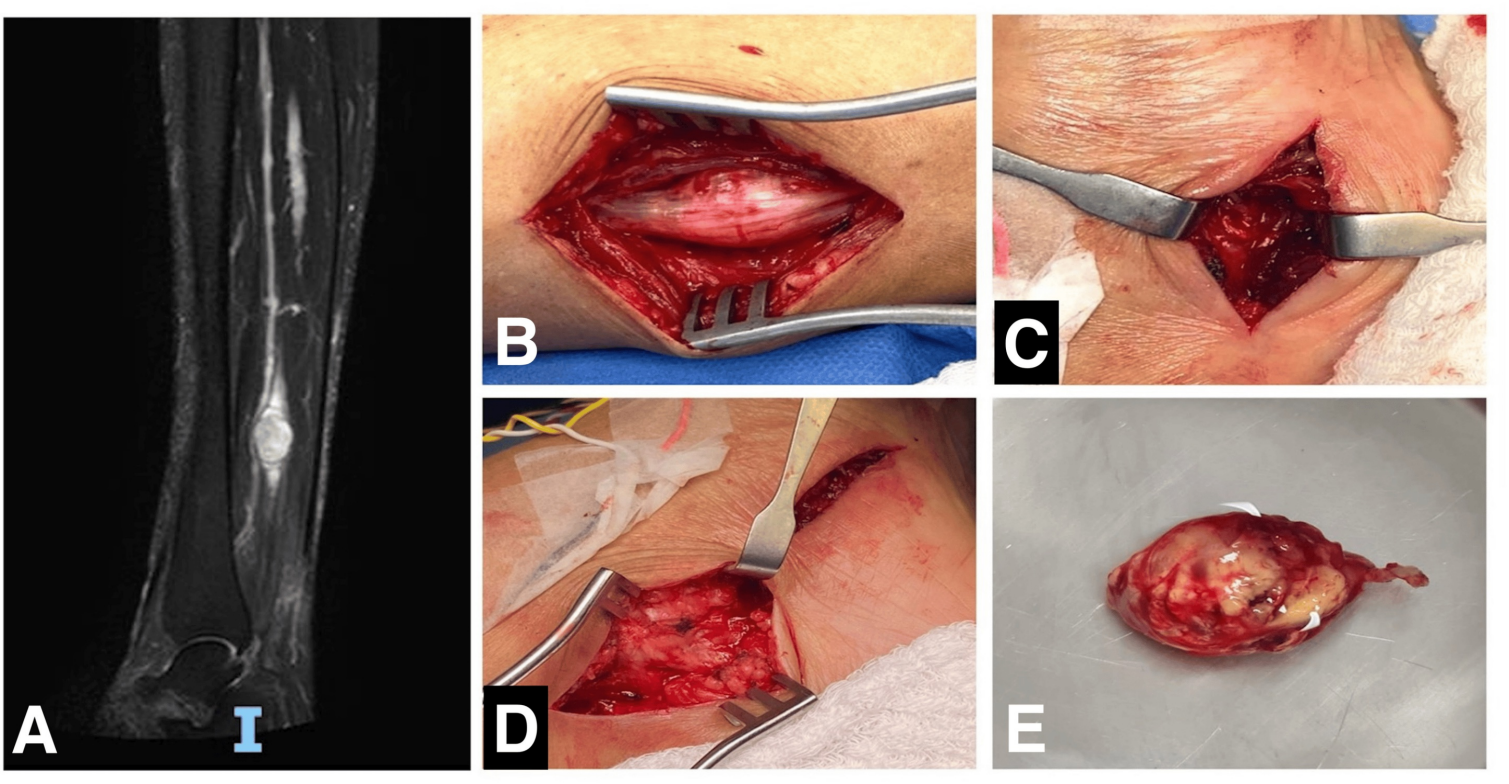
Case 2: Sagittal T2-weighted MRI showing a distal leg tibial nerve schwannoma (A); intraoperative views showing the tumor before gross total resection and complete tarsal tunnel decompression through two incisions (B-E). MRI, magnetic resonance imaging
